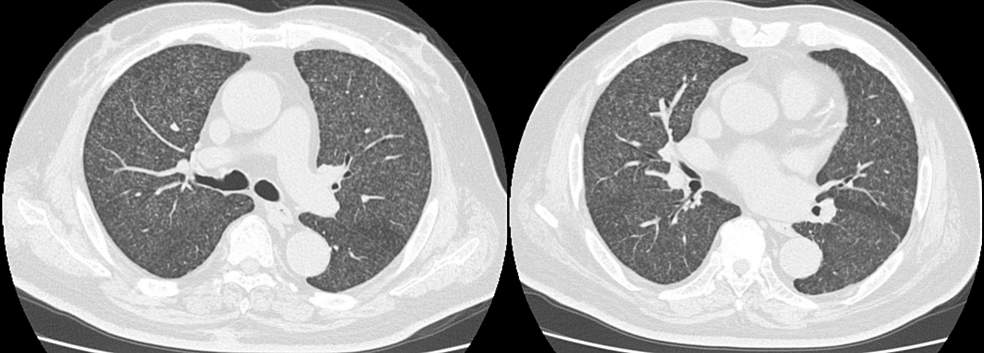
Chest CT Chest CT showing diffuse multiple tiny nodules in bilateral lungs.
